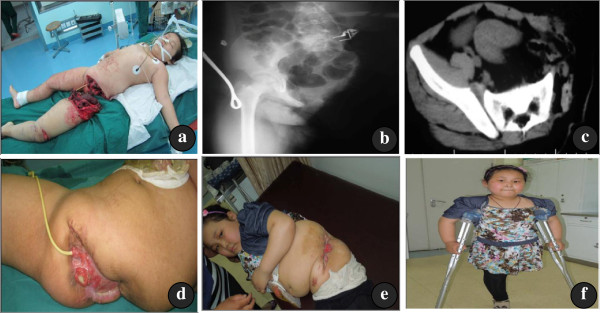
(a) A female, 7 years old, with complete hemipelvectomy caused by a traffic accident, referred to our hospital 6 h later. (b) The CT scan, and (c) X-ray film of the pelvis after the injury. Debridement was performed and the wound was left open. (d) By postoperative day 45, the majority of wound had healed. (e) &(f) The overview of the wound at the eighth postoperative month. The prolapse of the anal mucous membrane was noticed. The patient could ambulate with crutches.
